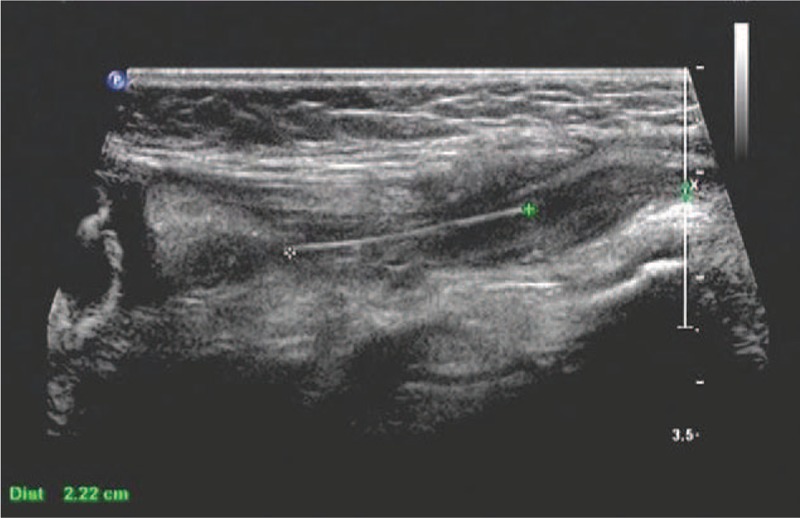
B-type US revealed a threadlike dense echo about 2.2 cm in the right groin. The ultrasound doctor considered that it was a sewing needle. US = ultrasonography.
